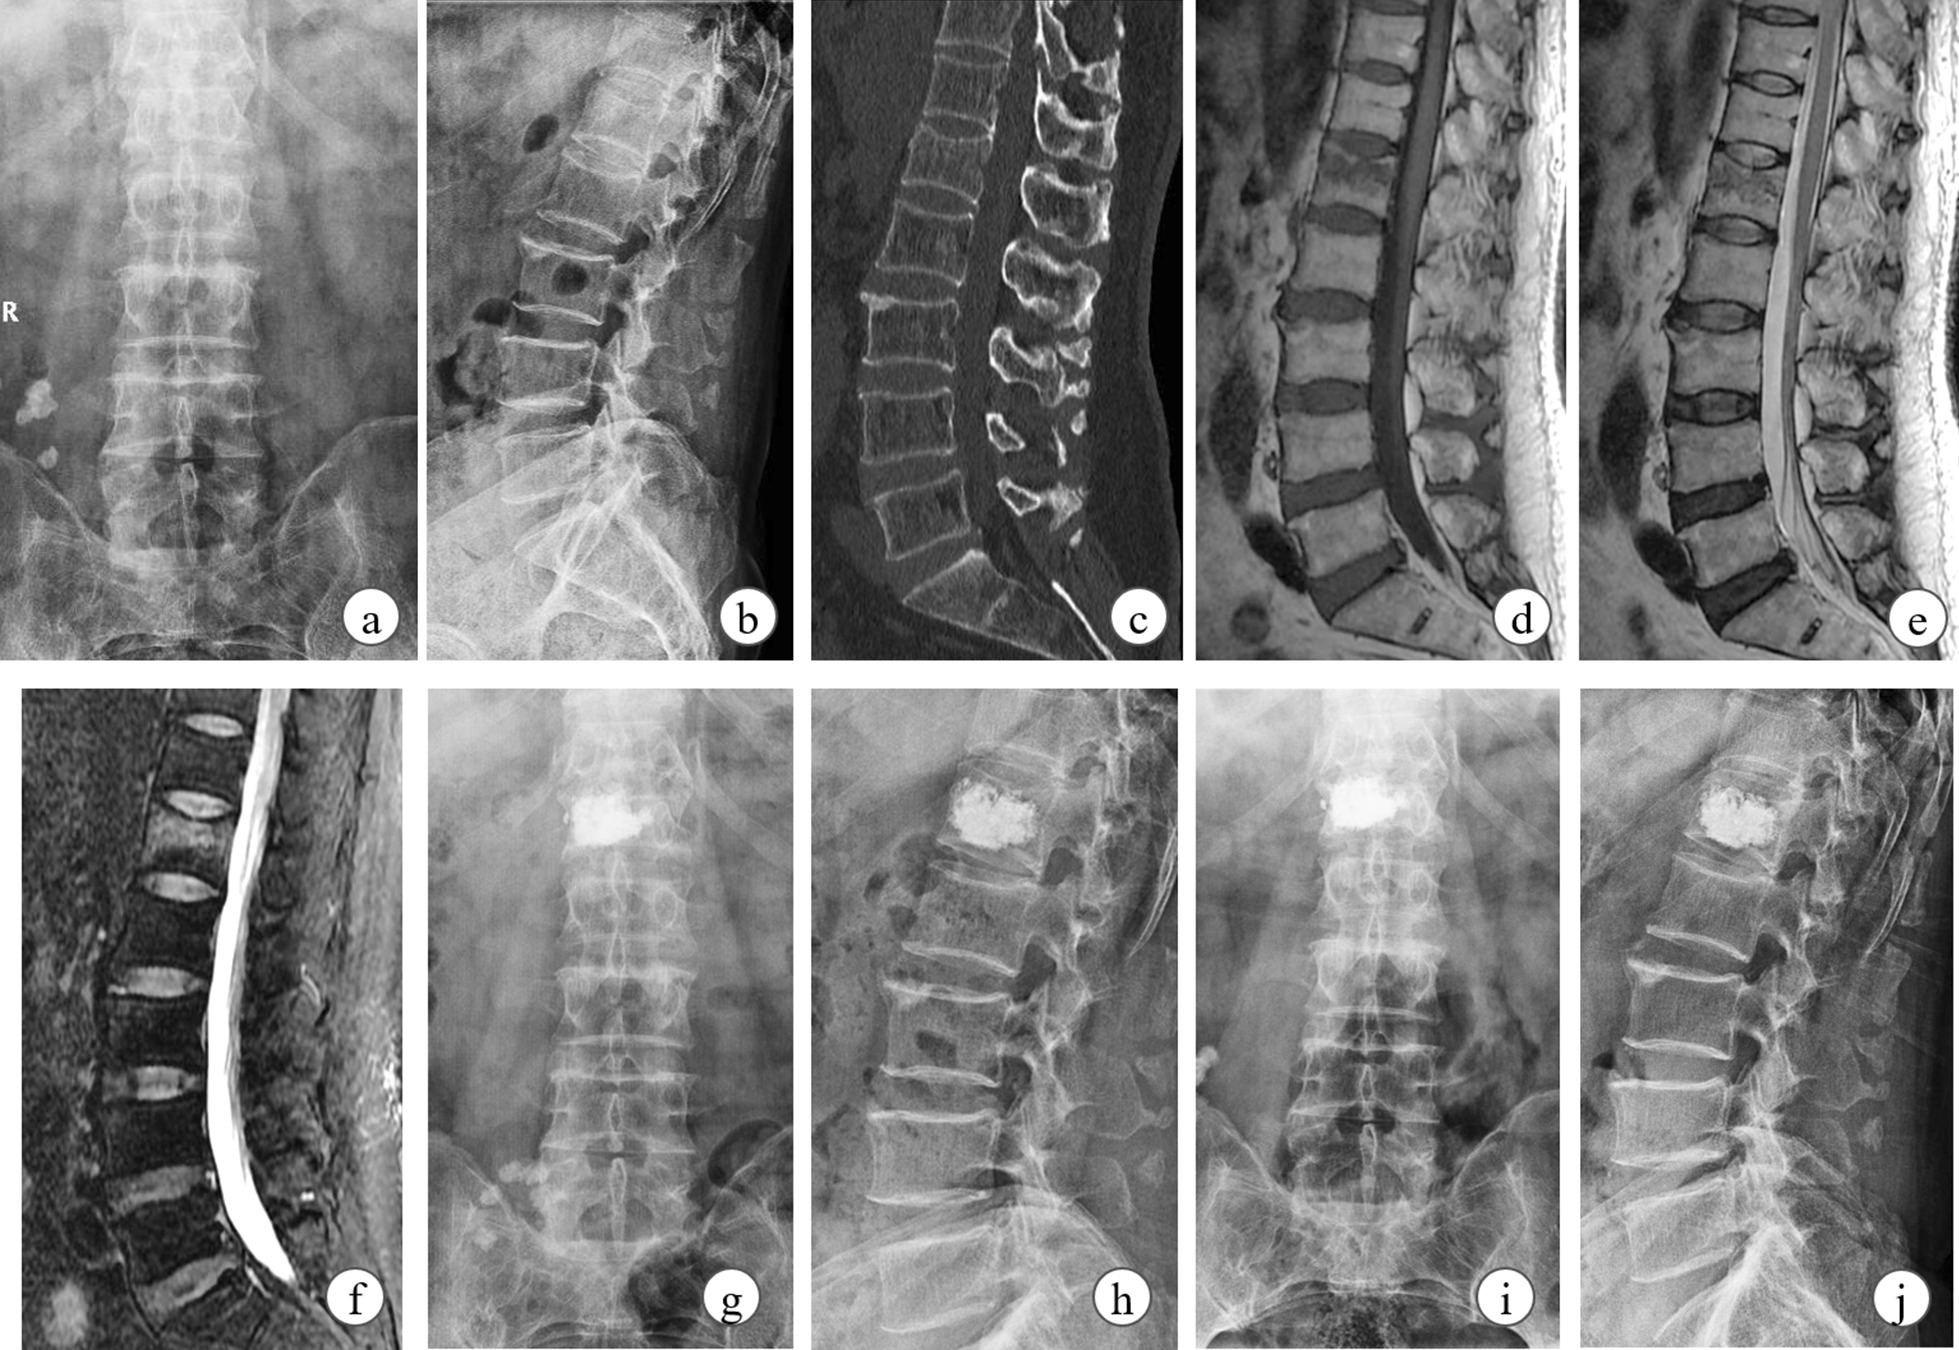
The clinical data of a 68-year-old male OVCF patient with UTP PKP surgery. The patient with the main complaint of severe back pain was diagnosed by radiographical examinations (af) as OVCF at L1 segment. At the first follow-up, cement was unilaterally distributed but vertebral height was almost restored (g, h). No further obvious collapse and symptom recurrence was noted at 1-year postoperative follow-up (i, j)
